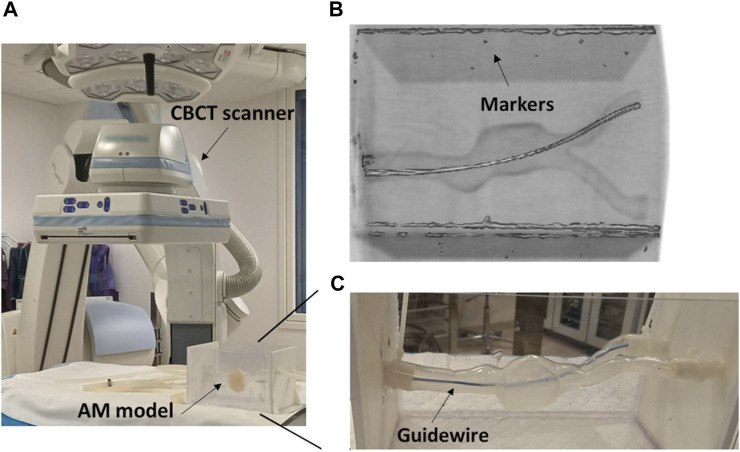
(A) Detail of the CBCT scanner in the hybrid operating room, with the model placed in a rigid box. (B) Example of a CBCT acquisition, with the reference markers used for registration. (C) Detail of the additive manufactured (AM) model (Model A) with the guidewire fully inserted.
